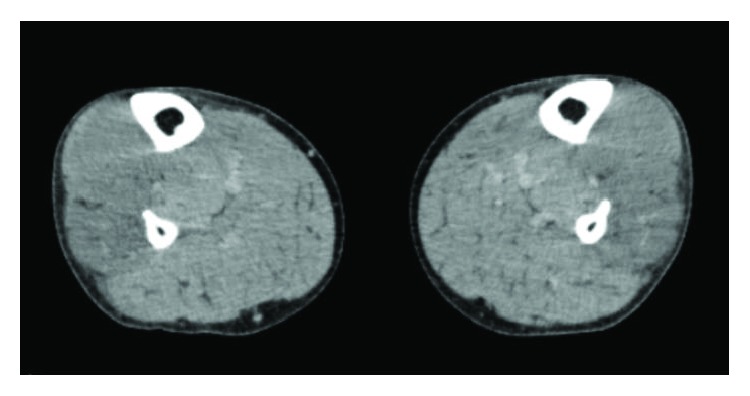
Contrasting computed tomography of the lower legs showed swelling and decreased enhancement of the muscles in the anterior and lateral compartments.
